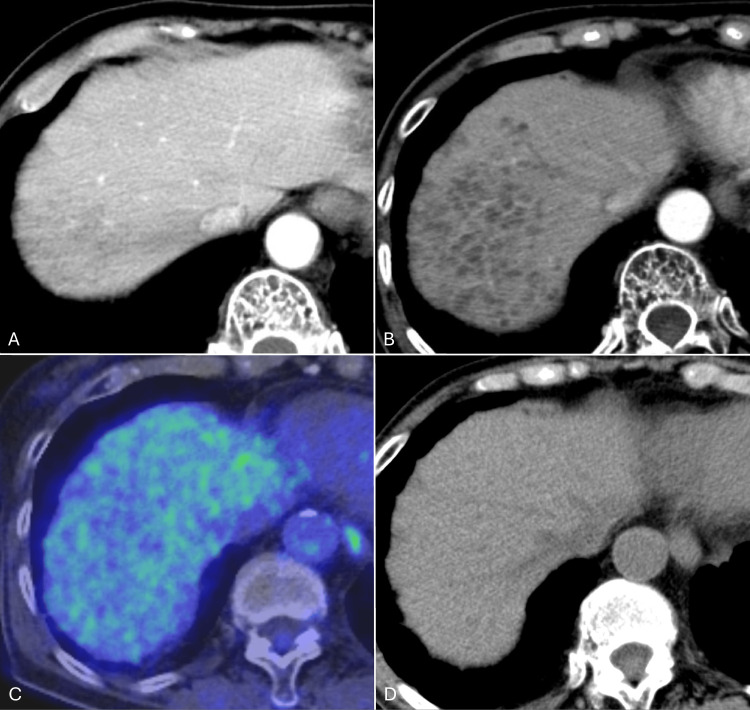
18F-FDG PET/CT and longitudinal CT images of peliosis hepatis A: Contrast-enhanced CT performed one year and four months ago showed multiple cystic lesions in the right hepatic lobe. B: Contrast-enhanced CT shows increased cystic lesions. C: 18F-FDG PET/CT shows decreased FDG-uptake areas in the lesions. D: Five months after the discontinuation of the suspected causative drug shows regression of the cystic lesions on nonenhanced CT. 18F-FDG PET/CT, 18F-fluorodeoxyglucose positron emission tomography/computed tomography
